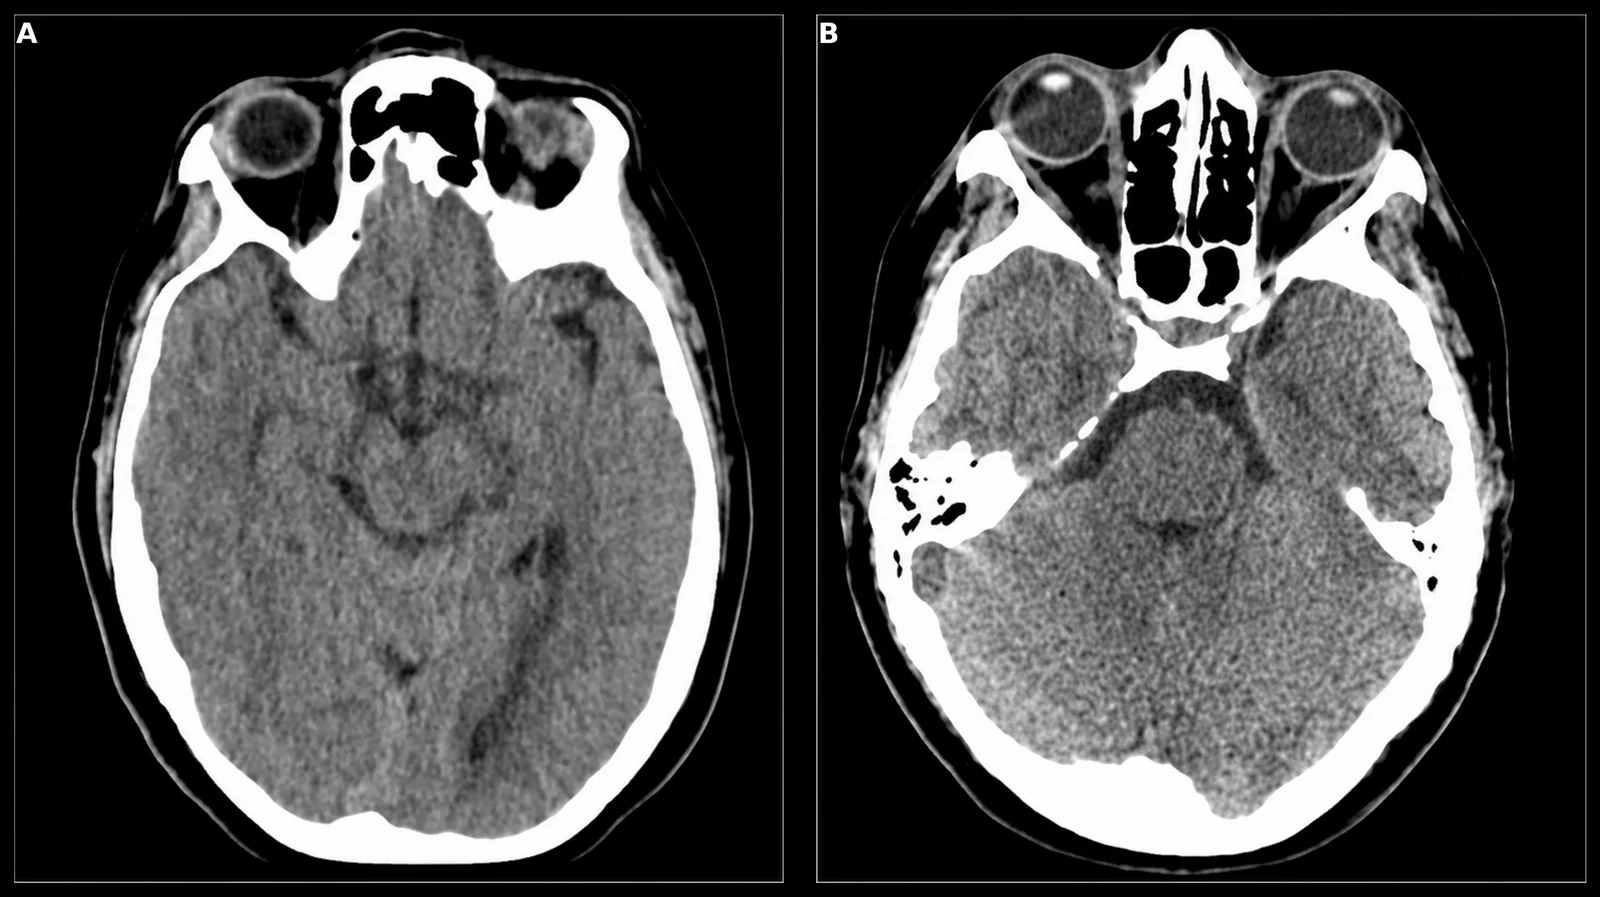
Serial non-contrast brain CT scans (A) Initial examination showing no acute intracranial lesion; (B) Follow-up examination performed 48 hours after admission showing no focal brain parenchymal density abnormality or significant interval change
